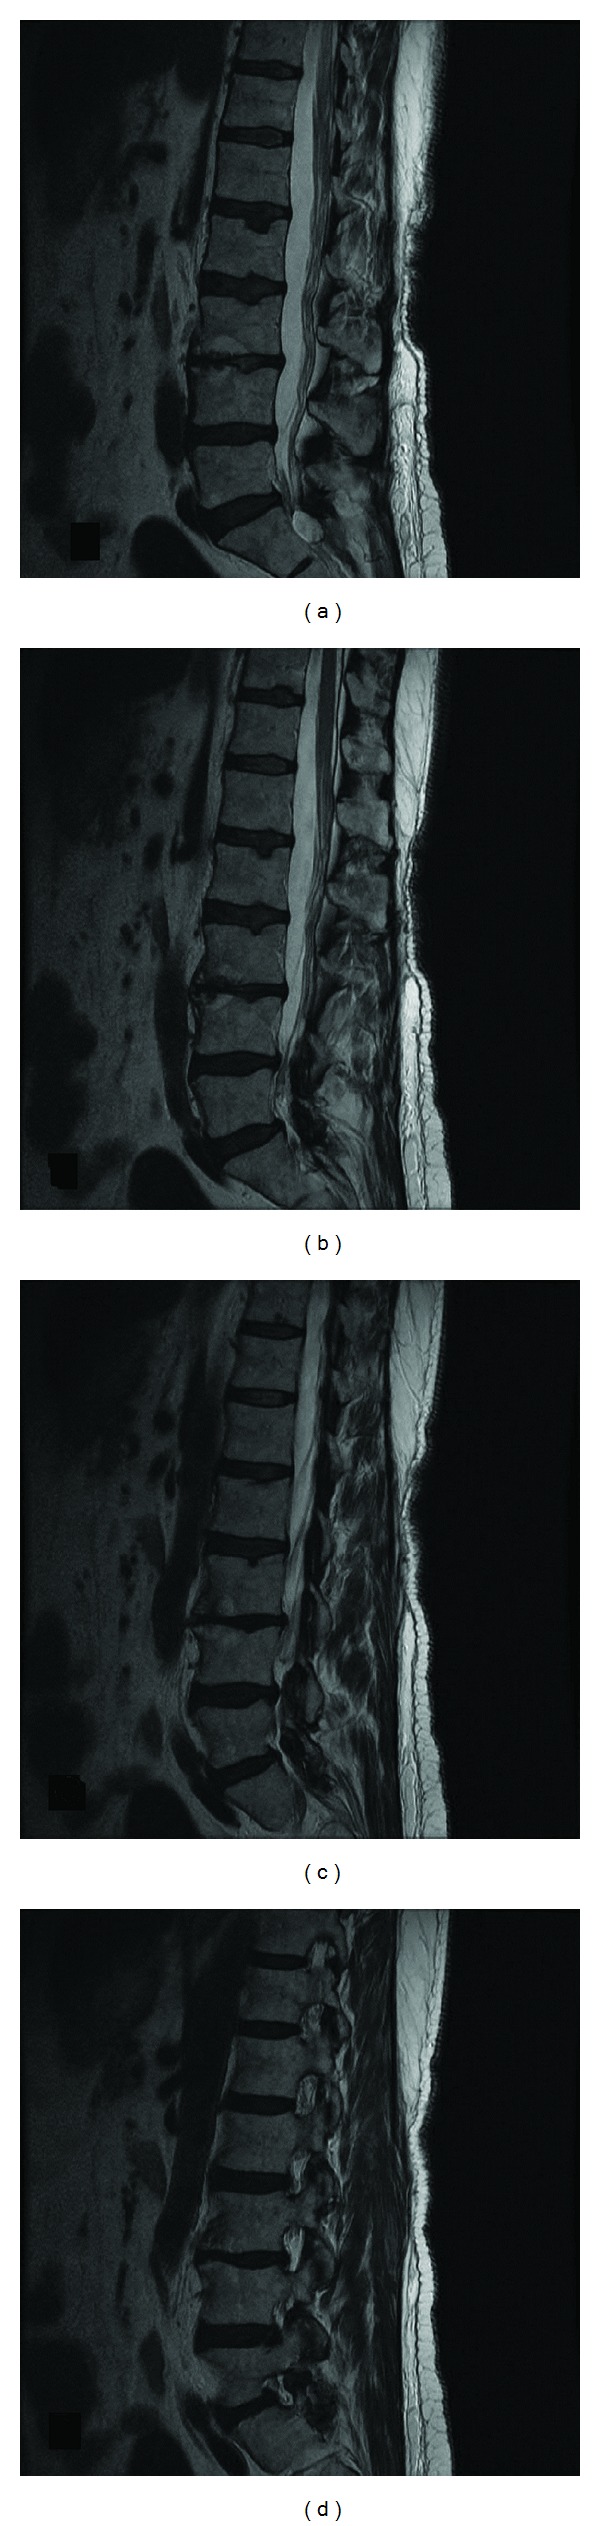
Sagittal T2-weighted MRI images showing sequential cuts ((a)–(d)) from midline to the foramen revealing the intrasacral mass separate from the left L5-S1 joint.
